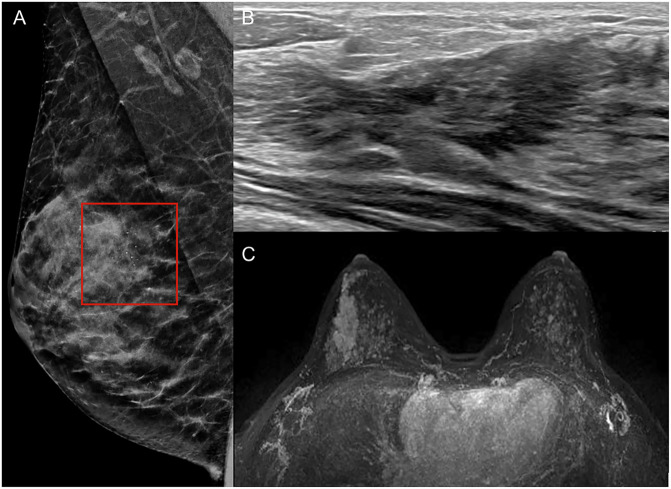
Fine pleomorphic regional calcifications (A, rectangle) observed in the upper outer quadrant of the right breast on mammography (BI-RADS 4a). B Corresponding hypoechoic non-mass with regional distribution is observed on US (BI-RADS 4a). C Segmental non-mass enhancement with heterogeneous enhancement is seen on MRI (BI-RADS 4a). Biopsy revealed fibroadenomatoid hyperplasia with non-specific granulomatous chronic inflammation (B2)
